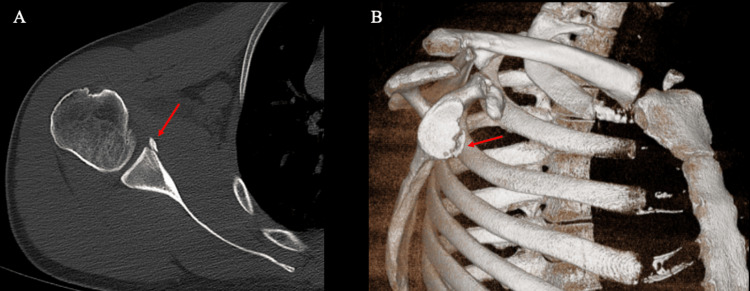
CT in the axial plane of the shoulder (Case 1) (A) glenoid fracture and bone loss, and (B) a 3D reconstruction indicating of the shoulder demonstrating anteroinferior glenoid fracture and bone loss. CT: computed tomography; 3D: three-dimensional
